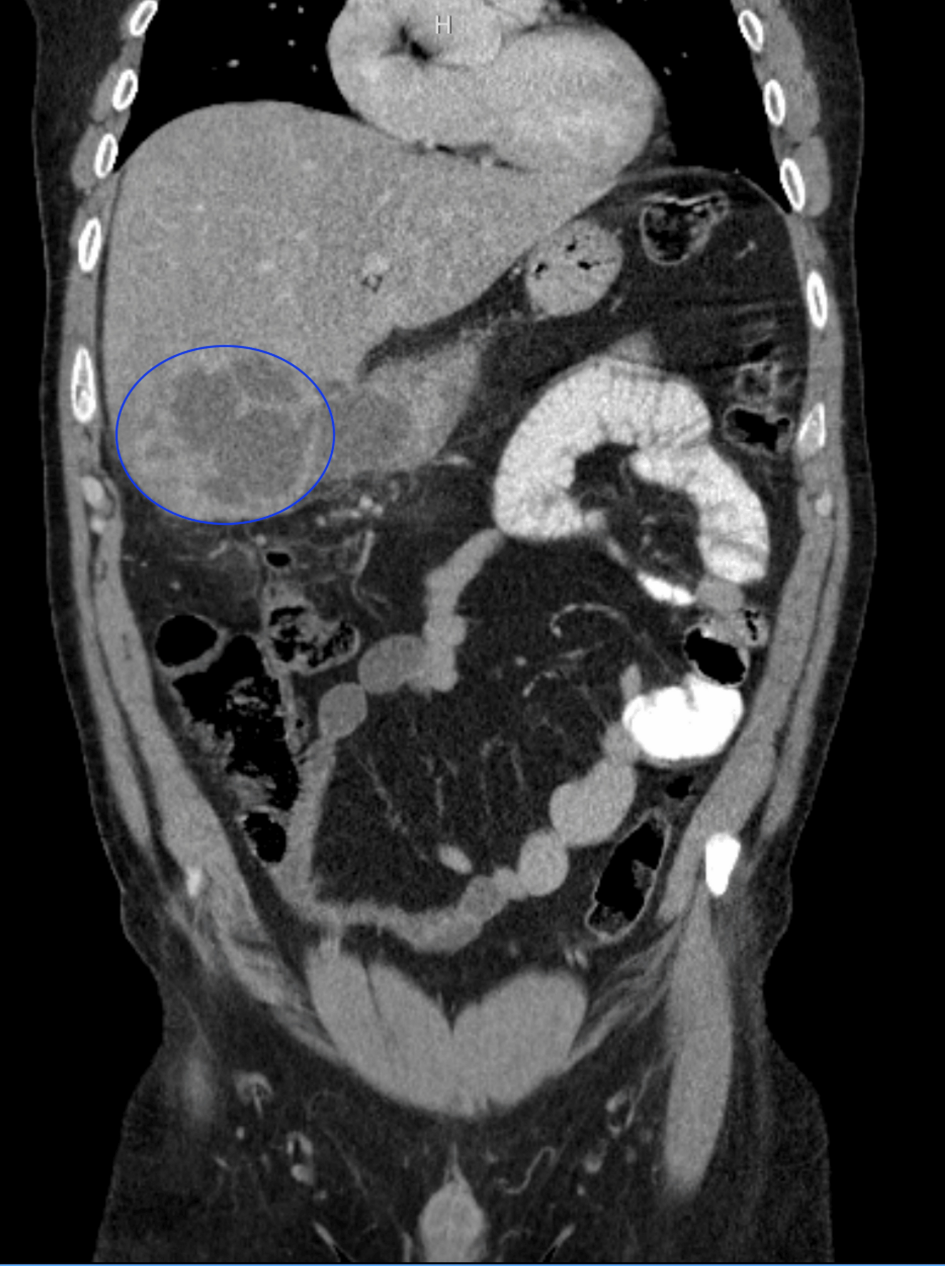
Hepatic abscess found on CT abdomen/pelvis with IV contrast on admission (blue circle)
